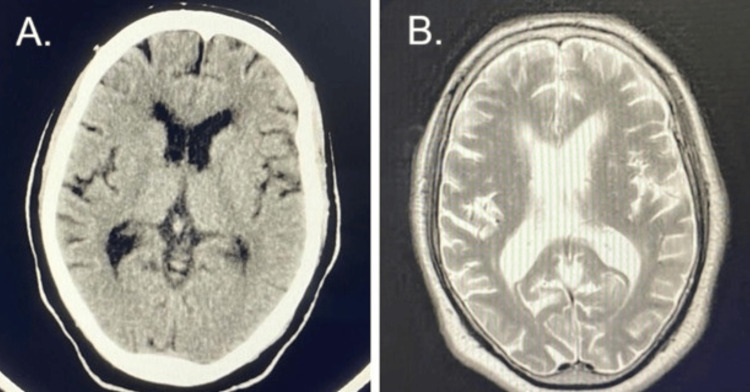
(A) Non-enhanced CT and (B) T2-weighted MRI of the brain in a hemodialysis patient CT, computed tomography; T2WI, MRI, magnetic resonance imaging
